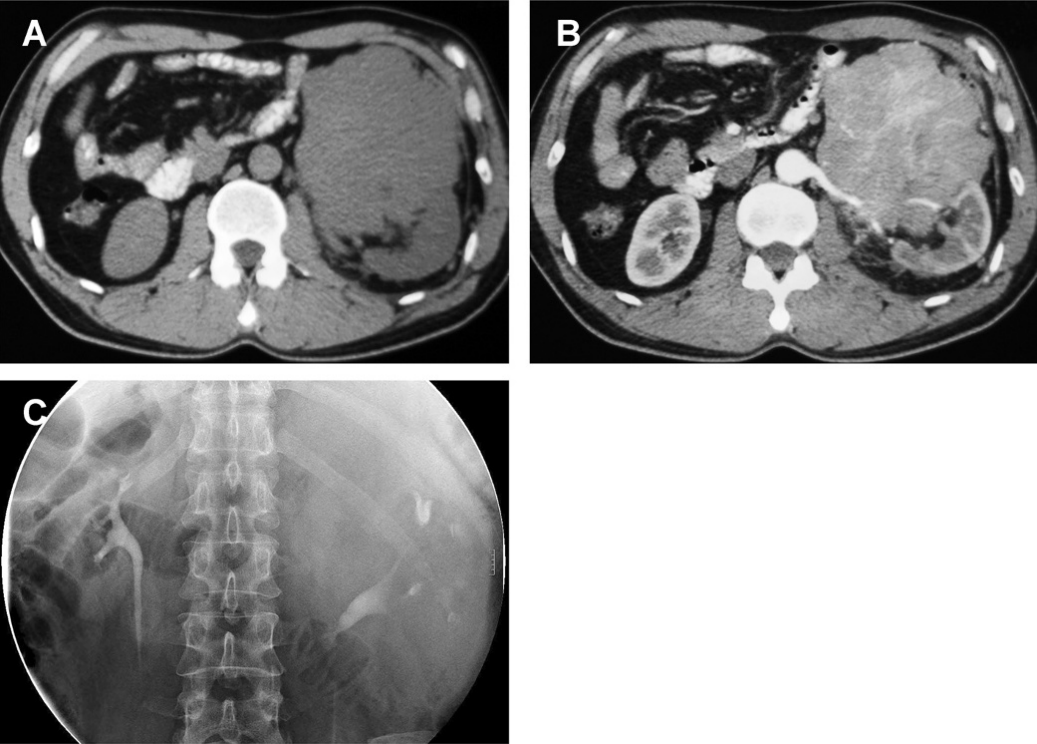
CT scanning and intravenous urography show the mass in the left renal hilum. The tumor displaced tissue near the renal hilum, and invaded the parenchyma and renal vessels. A: The plain scanning of the tumor. B: The contrast-enhanced phase of imaging. C: The collecting system of the affected kidney was compressed and distorted.
